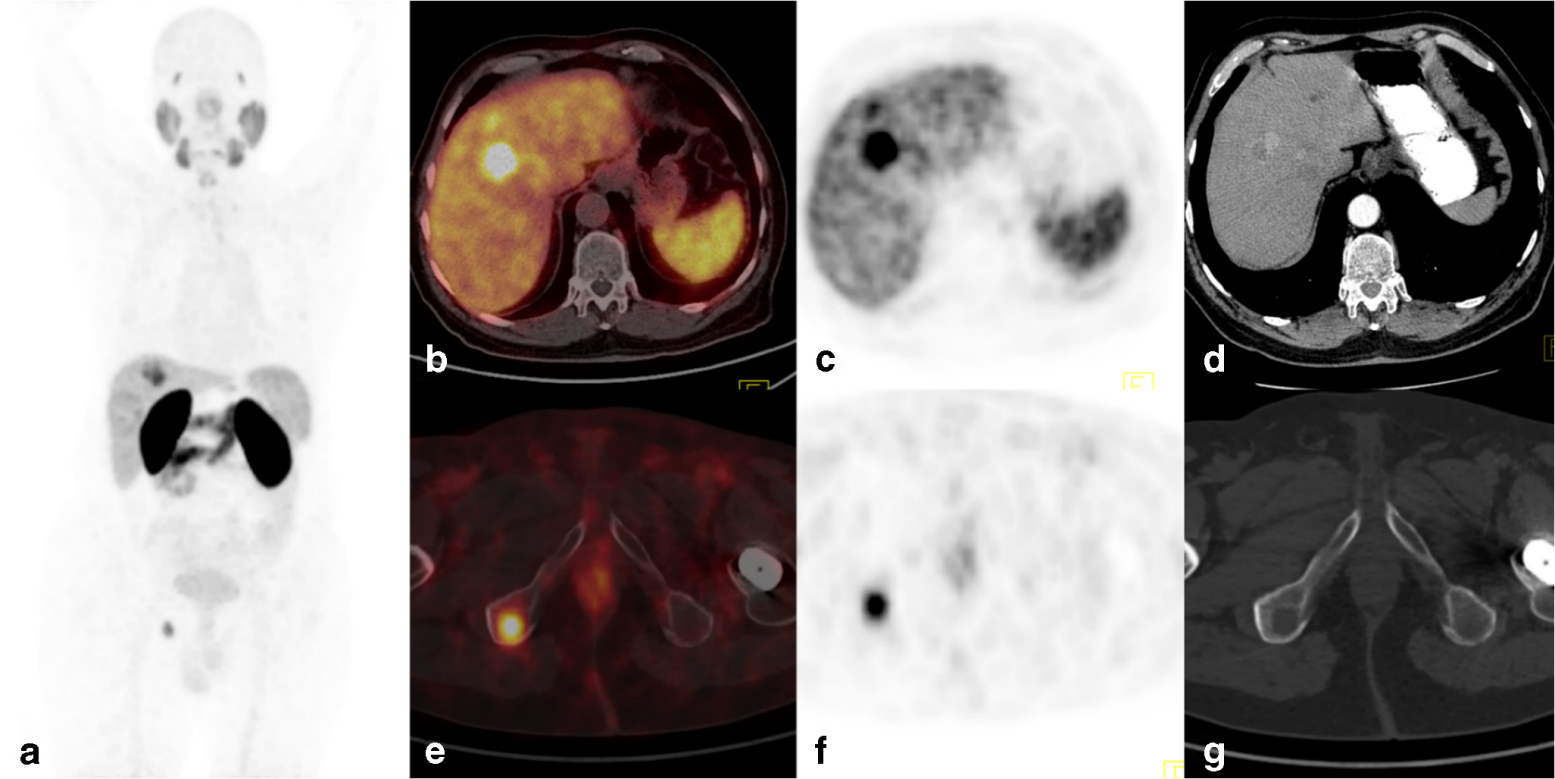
[^68^Ga]Ga-PSMA-11 PET/CT images of a 66-year-old man with hepatitis B virus (HBV) infection (patient no. 10). Follow-up revealed elevated CEA and AFP levels. On CT, there were two lesions suspicious of HCC. The [^68^Ga]Ga-PSMA-11 PET/CT images showed multiple liver tumors (SUV_max_ 7.4–17.8) and distant metastases in the bones (right VI rib, SUV_max_ of 2.2; right ischium, SUV_max_ of 12.0). a Maximum intensity projection image (MIP). b, e CT. c, f PET. d, g Fused axial PET/CT. Core needle biopsy of the liver revealed hepatocellular carcinoma grade 2. The patient was disqualified from surgery
